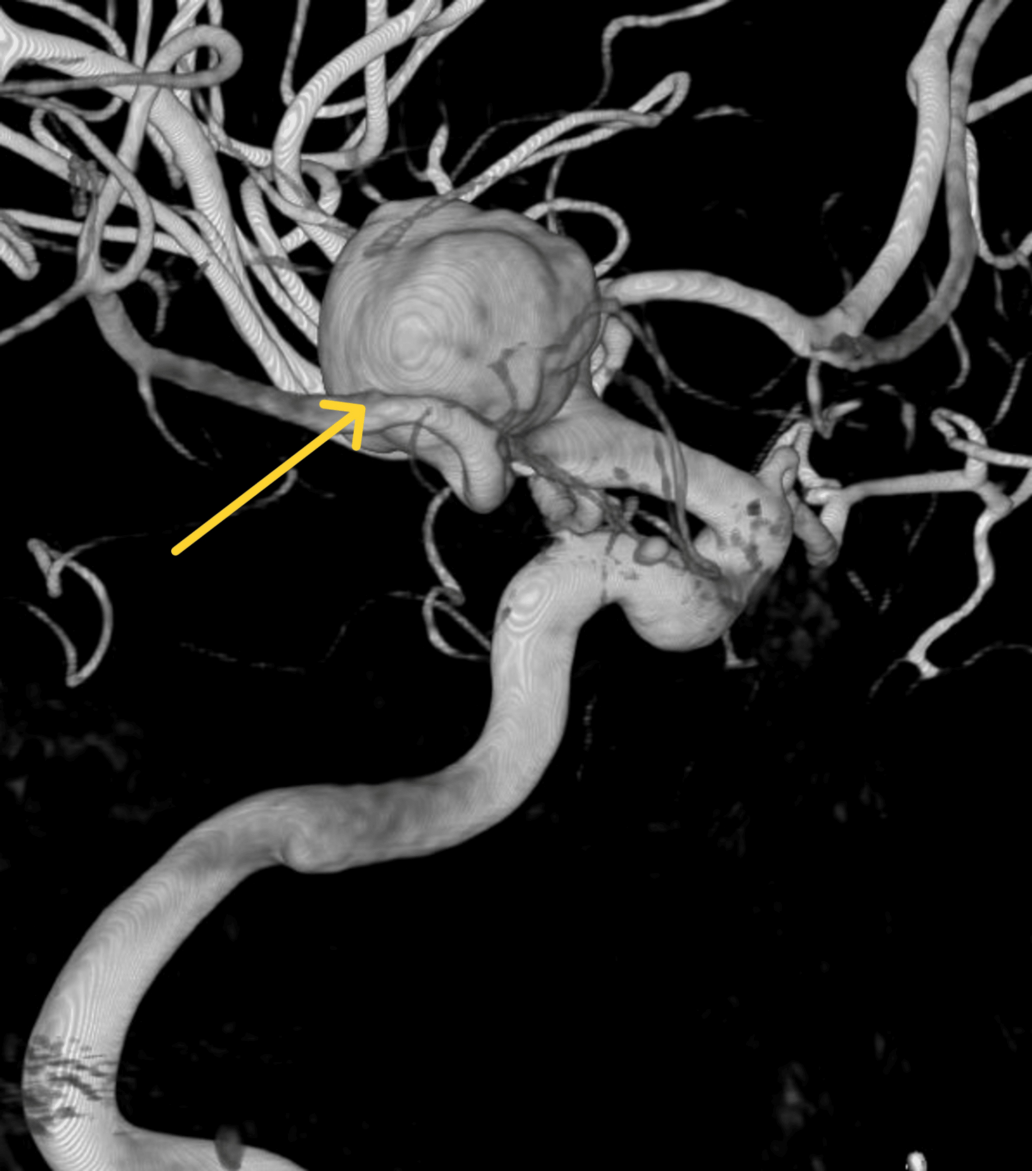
3D Computed tomography angiography, showing large aneurysm in the left posterior communicating artery.
